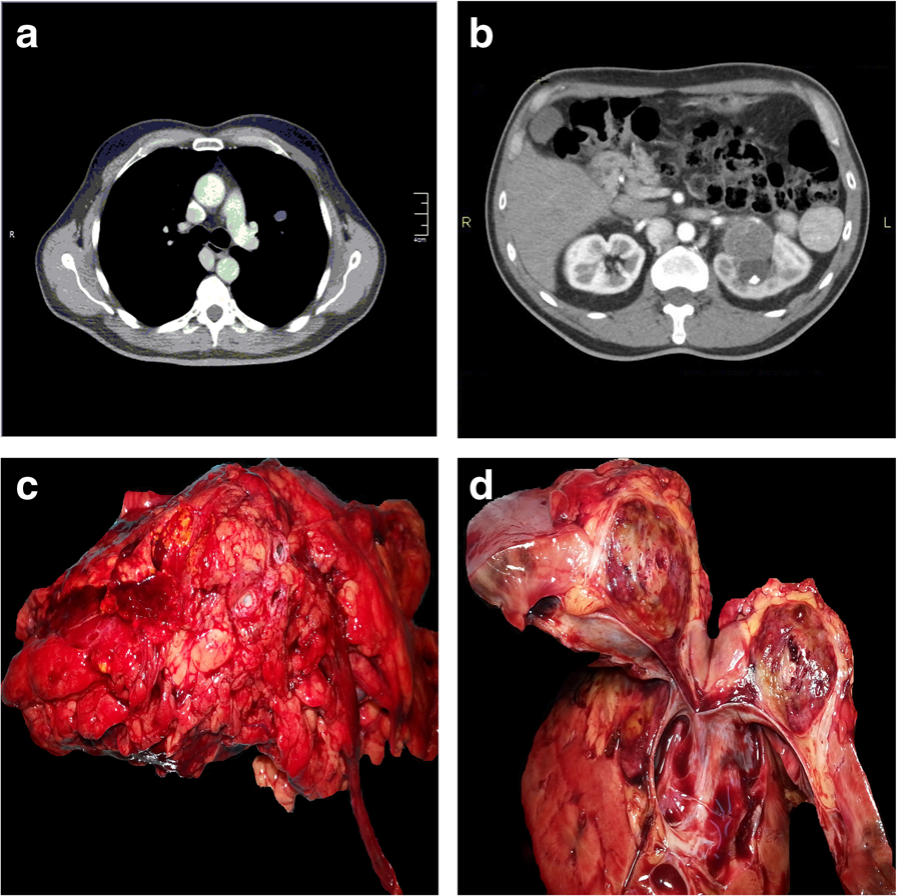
a Computed tomography: imaging identifies an intraparenchymal nodule in the left lung. b Computed tomography: imaging identifies the presence of a nodular mesonephric hypodense lesion characterized by post contrastographic enhancement. c Gross imaging: the kidney shows an irregular profile with granulating appearance of the hilum. (d) Gross imaging: sectioned tumor appears hemorrhagic, soft, and reddish
